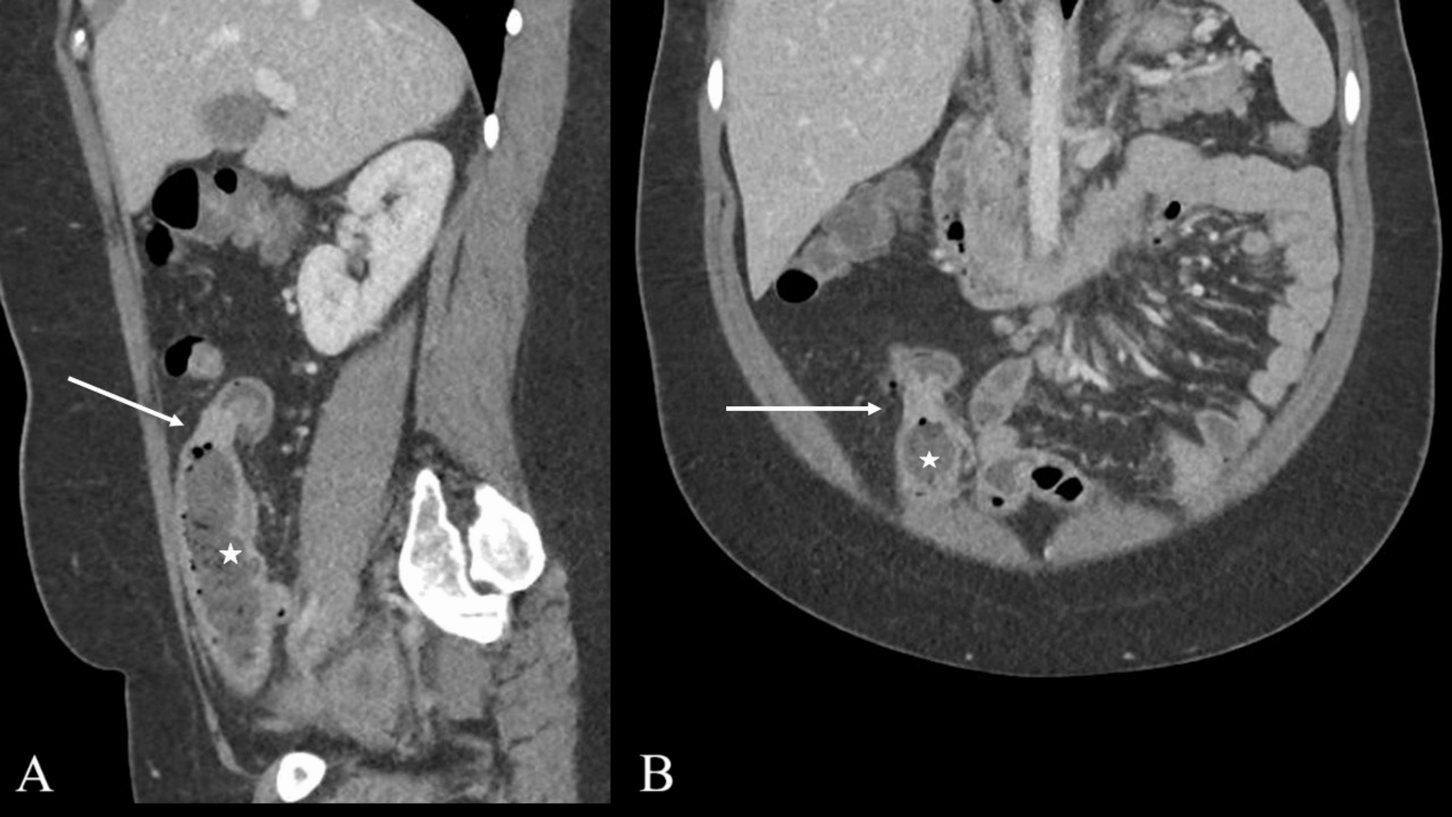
Abdominal tomodensitometry. Sagittal (A) and coronal (B) views of the abdominal CT after intravenous contrast administration showing a circumferential stenotic ileal wall lesion (arrows) with upstream small bowel dilation and a feces sign, indicating delayed transit.
